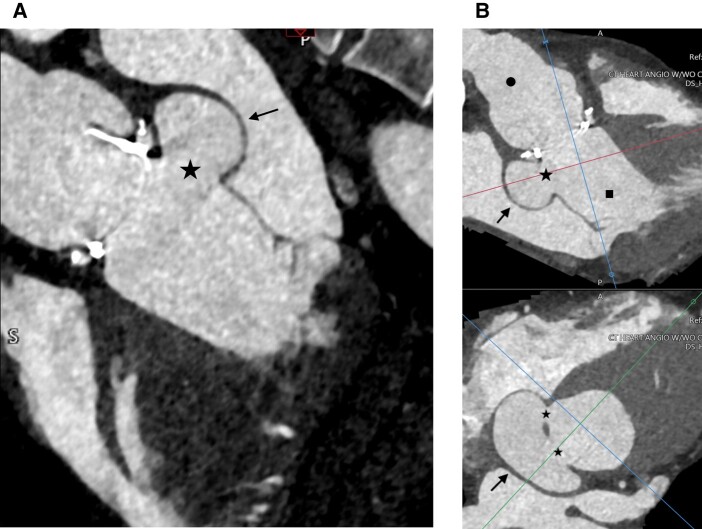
Cardiac computed tomographic angiography imaging. Pseudoaneurysm (arrow) can be seen on the medial aspect of the left ventricular outflow tract body measuring 4.4 cm × 2.2 cm (*A*). The stars indicate the openings to the pseudoaneurysm. The patient’s bioprosthetic aortic valve can be seen just superior to the pseudoaneurysm. Additional views include CCTA showing two openings into the pseudoaneurysm and the corresponding orthogonal plane through each opening into the pseudoaneurysm (*B*), with ascending aorta (circle) above this, and left ventricular outflow tract (square) below.
